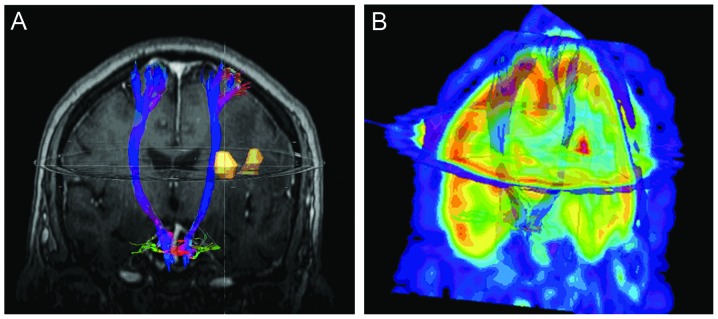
BrainLAB planning software was used to reconstruct the corticospinal tracts (CST) and reveal the three-dimensional (3D) anatomical association between the CST and the brain lesions, according to the ^18^F-fluorodeoxyglucose-positron emission tomography (PET) and contrast-enhanced magnetic resonance imaging (MRI) fusion images. (A) MRI-diffusion tensor imaging (DTI) fusion image revealing the 3D association between the lesion and the CST. (B) PET-DTI fusion image revealing the 3D association between the lesion and the CST.
